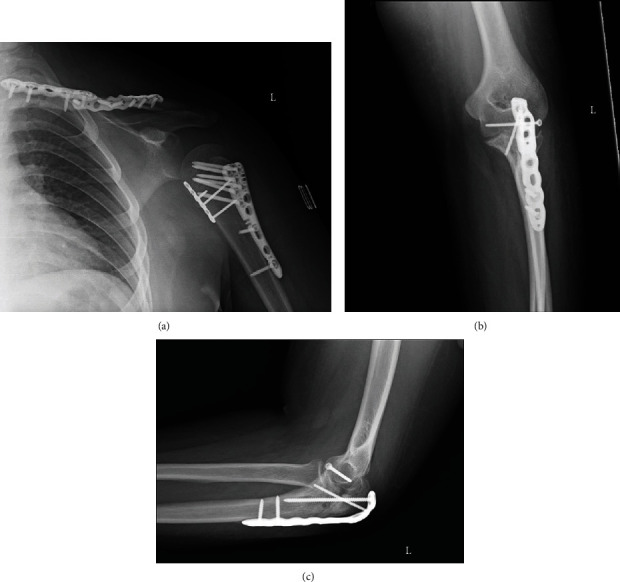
(a) Anteroposterior X-ray of the left shoulder, (b) anteroposterior X-ray of left elbow, and (c) lateral X-ray of the left elbow reveal progressive healing of fractures after six weeks postsurgical intervention.
